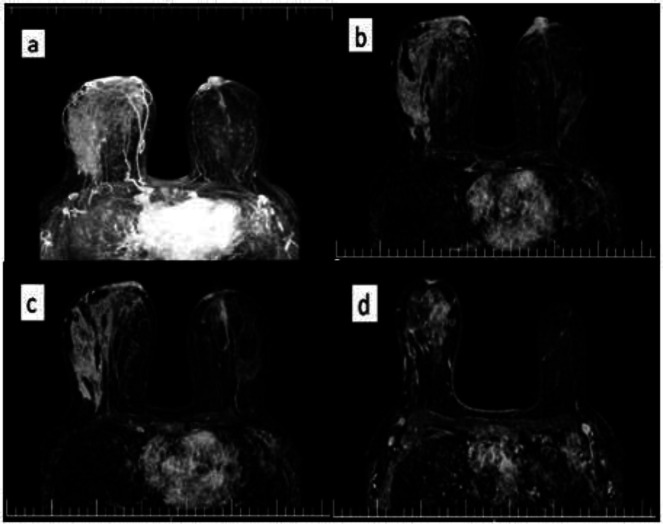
MRI of breasts and both axillae with pre contrast axial T1 and T2w image technique. a. MRI / MIP subtraction images: extensive enhancement of right breast in lateral and anterior portion + enhancement and enlargement of left nipple along with areolar skin enhancement linear non mass enhancement at central retro- nipple. b, c & d: MRI subtraction images significant regional / multiple regions of enhancement in right breast and significant enlargement + enhancement of left nipple associated with thickening and focal enhancement of areolar skin
